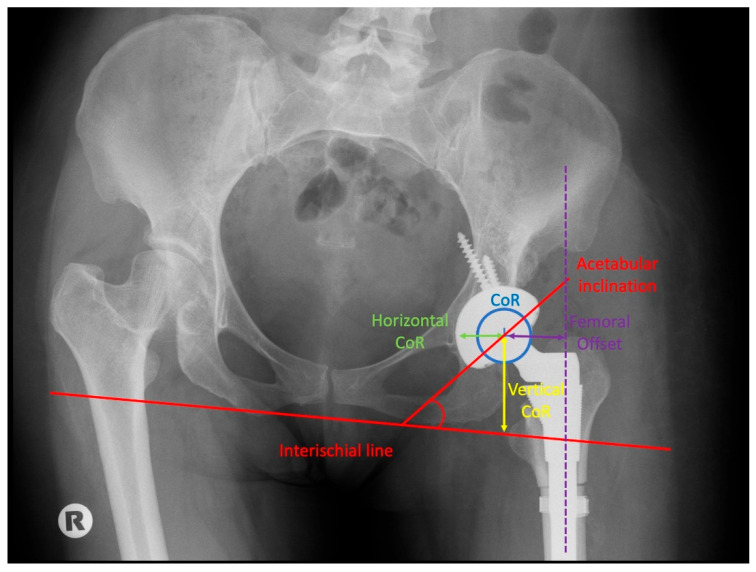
Postoperative radiographic assessment of implant position: horizontal and vertical centre of rotation, acetabular inclination, and femoral offset.
